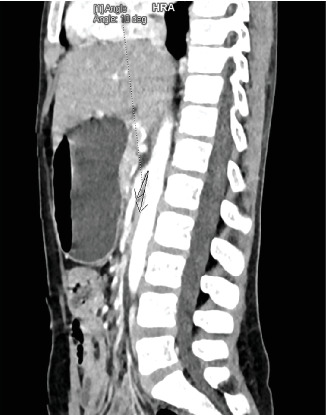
Abdominal CT scan showing narrow AMA (10°).
